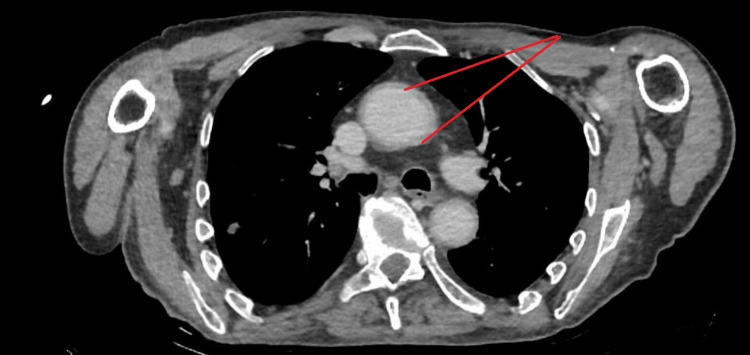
CT aortogram Red arrows point to circumferential aorta thickening
